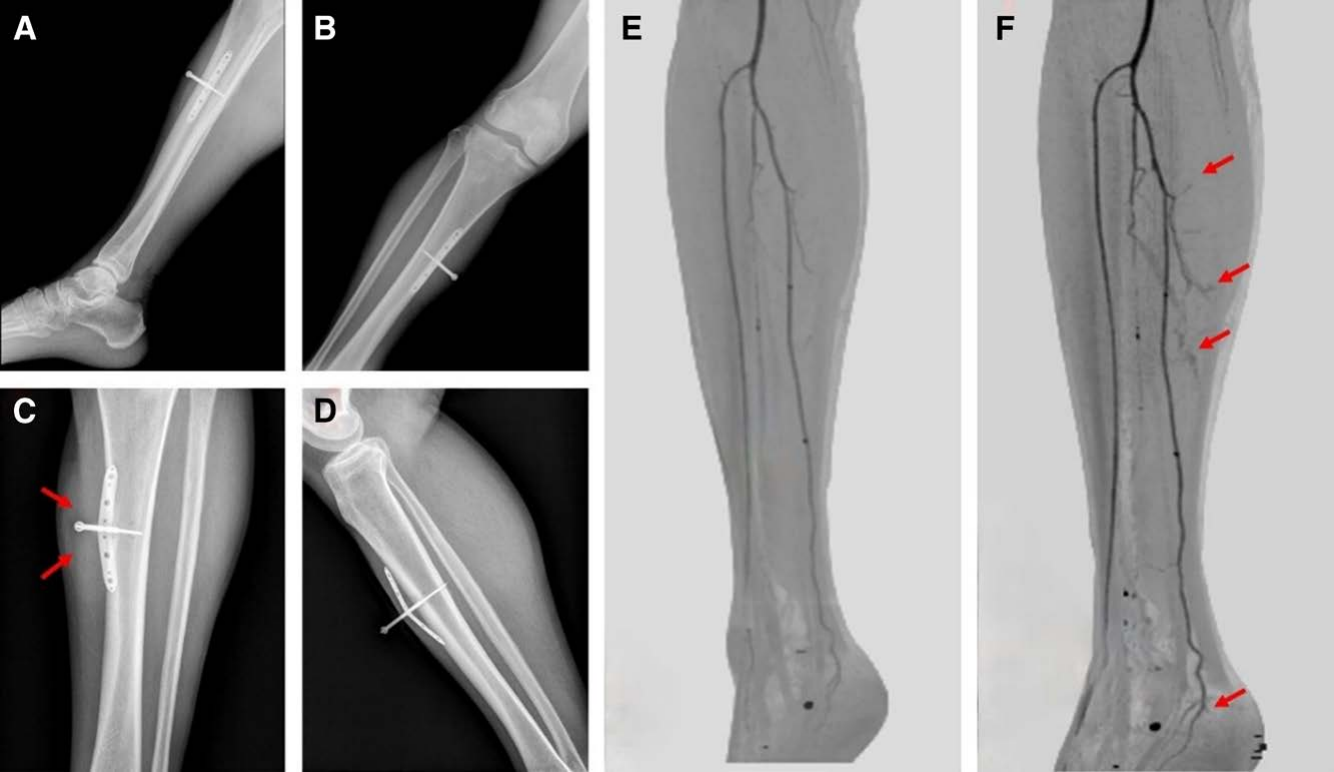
Periosteal distraction process and CTA vascular visualization of the affected limb before and after surgery. (A and B) refers to the DR condition of the affected limb on the first day after periosteal distraction, periosteal distraction plate is located in the middle and upper tibia, abutting the bone surface; (C and D) refers to the DR condition of the affected limb on the 14th day after periosteal distraction, periosteal distraction plate has bulged the bone surface with the continuous rotation of cannulated screws, so that the periosteum is continuously distracted, (C) (red arrow) showed soft tissue thickening, and inflammatory shadows; (E and F) refers to the CTA vascular development contrast of the affected limb before surgery (E) and 3 mo after surgery (F), in (F) (red arrow) showed that the vascular development of the affected limb 3 months after surgery has significantly increased and thickened compared with that before surgery, and formed an interwoven network, meanwhile, the closer to the periosteal distraction site, the denser the neovascular network, also a few neovascular shadows were also observed in the heel (red arrow). CTA = computed tomography angiography.
